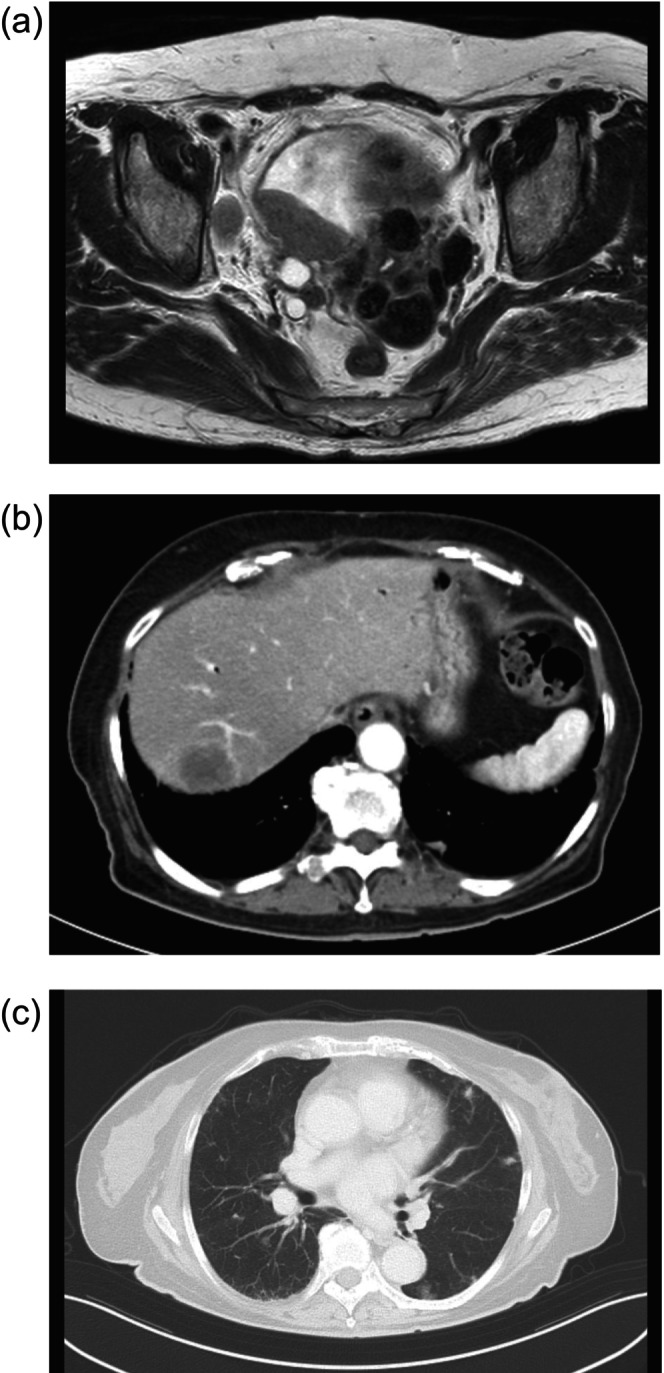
Computed tomography (CT) scan imaging at the time of diagnosis. The patient had massive bladder cancer with multiple lymph nodes, lung, and liver metastases. (a) In the pelvis. (b) In the liver. (c) In the lungs.
